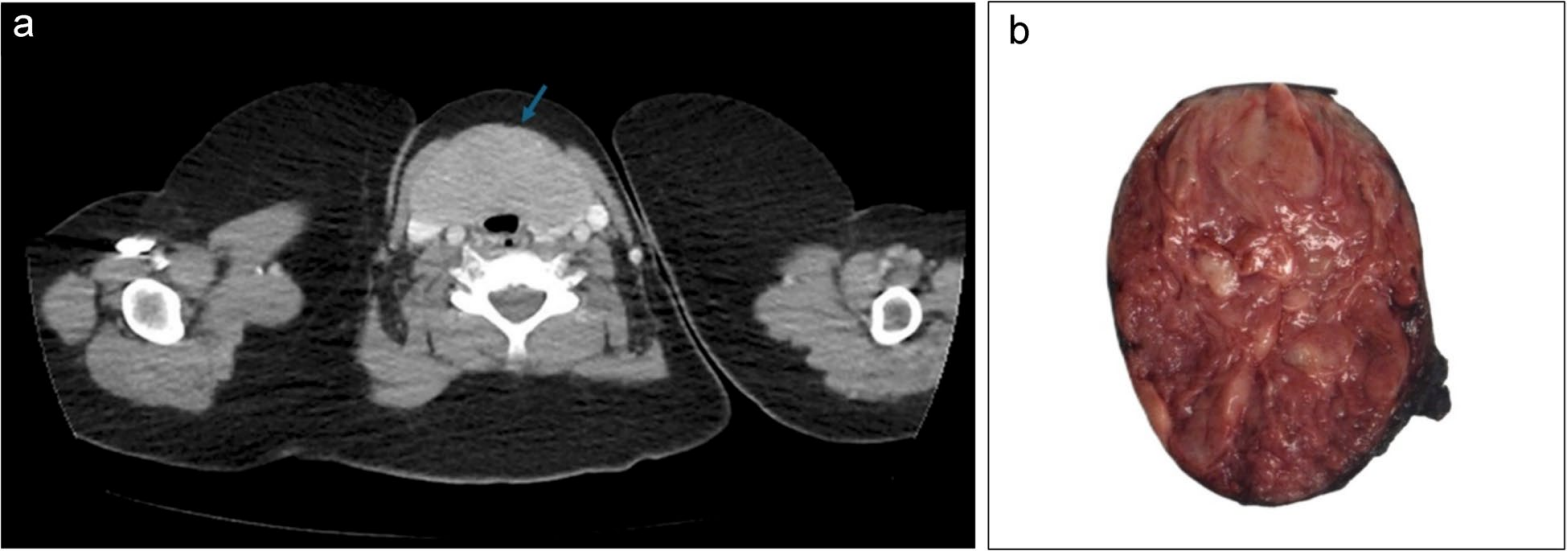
Patient 1. a Contrast-enhanced CT scan demonstrating diffuse thyroid involvement extending across the midline (arrow). b Gross photograph of the cut surface showing an expansile, well-demarcated, lobulated tan-brown mass confined to the thyroid gland
